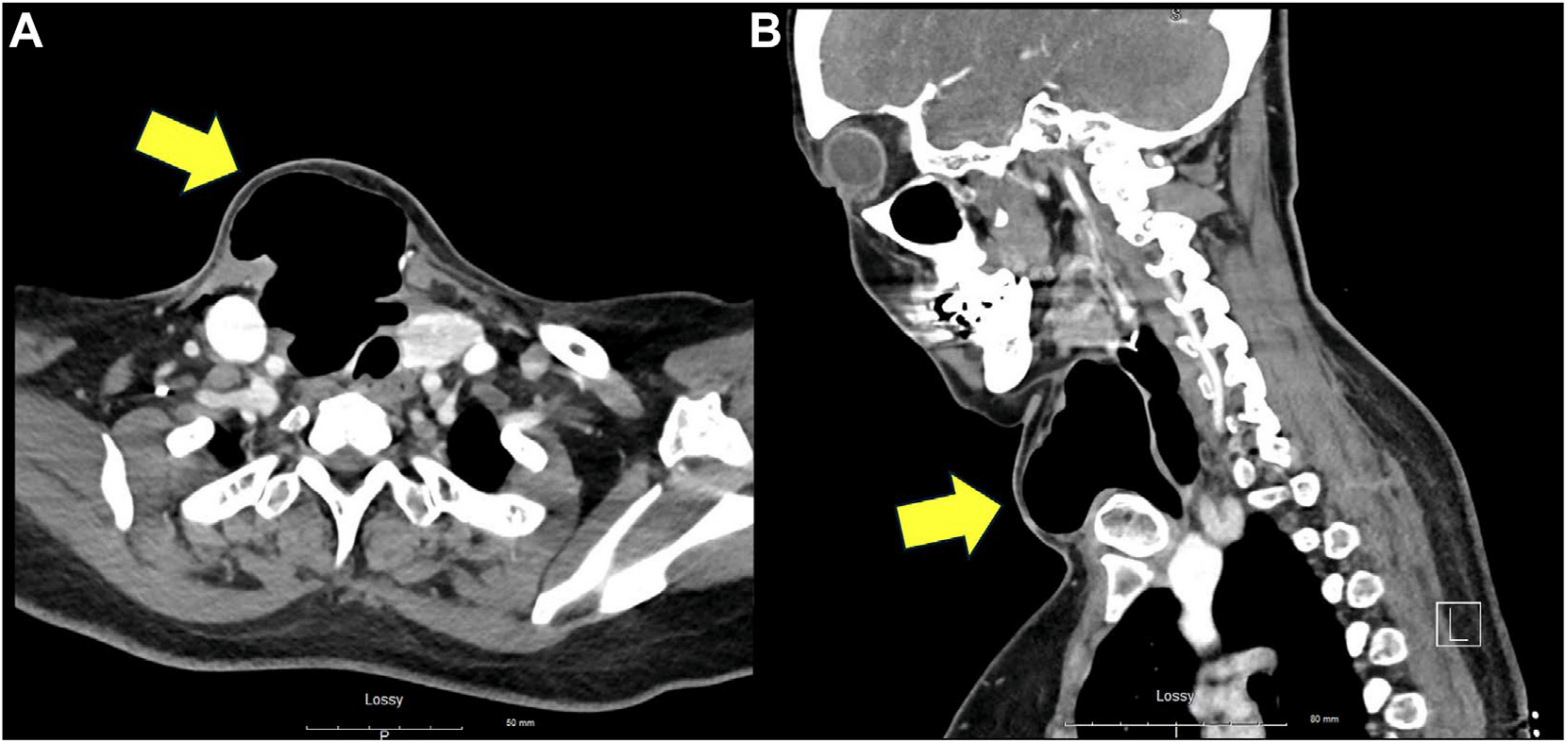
Axial (*A*) and sagittal (*B*) CT neck images showing a 9.5 × 7.0 × 6.0 cm air collection (yellow arrows) in right thyroidectomy bed. *CT* = computed tomography.
